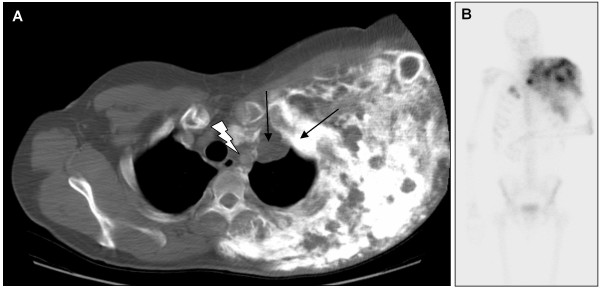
Radiological features 4.5 years after the first symptoms. (A) CT scan: thoracic transversal view showing a voluminous heterogeneous highly calcified mass. It included the whole left chest wall and the left scapula, with intercostal invasion to the apical pleura (black arrows) and the antero-superior mediastinum (bolt) with trachea deflection to the right. (B) Bone scan with Technecium 99: see the heterogeneous extended hyperfixation of the whole left side of the chest. There was a muscular extension to the triceps leading to a frozen scapulo-humeral joint.
